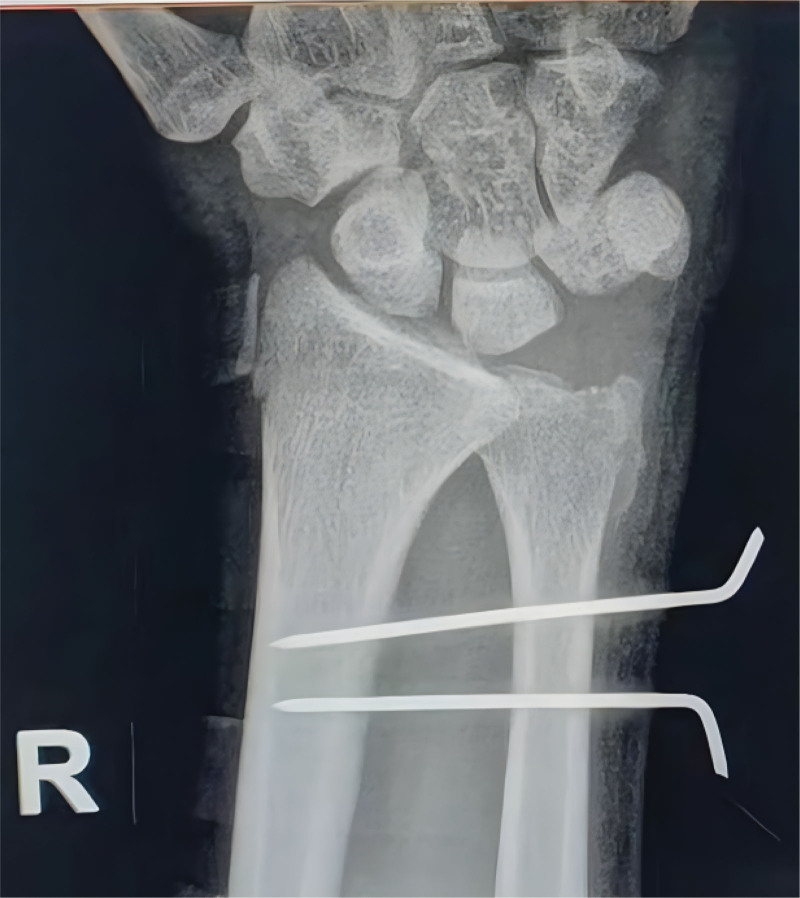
Posteroanterior radiograph after the initial surgery.
